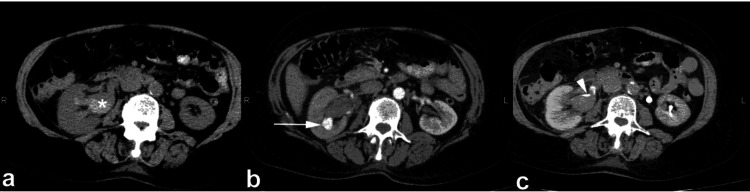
Non-enhanced CT (NECT) (a) is followed by a contrast-enhanced CT (CECT) scan of the artery (b), and subsequently, the urographic phase (c). In these sequences, an arrow highlights a contrast-filled outpouching detected within a branch of the right renal artery. Notably, blood is observed to be draining into the renal pelvis (asterisk), indicating the presence of a pseudoaneurysm with a fistula in the pyelocaliceal system. Additionally, attention should be paid to the iodinated contrast media that have been flushed out in the anterior portion of the renal pelvis (arrowhead), accentuating the anatomical details and aiding in the characterization of the vascular anomaly. This figure is a republished image, permission was obtained from the original publisher, https://epos.myesr.org/poster/esr/ecr2018/C-2802
